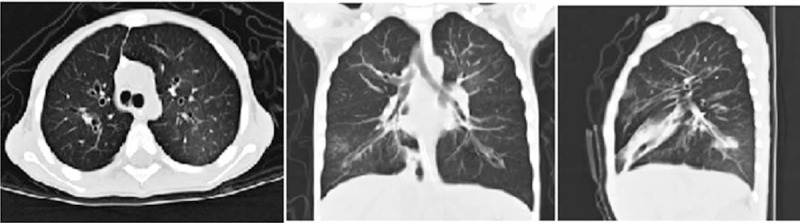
HRCT image showing bilateral bronchi and their branches locally dilated and wall thickened.
